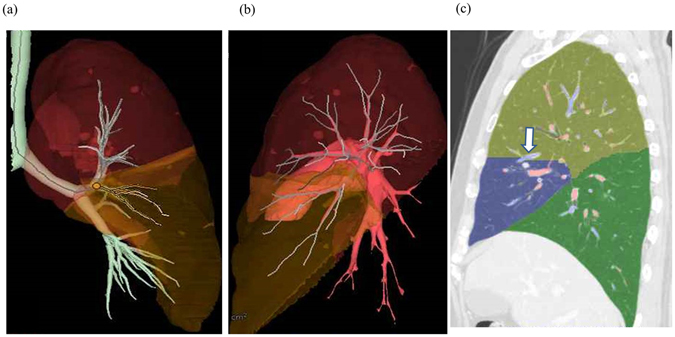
(a and b) According to the 3D-CT images, lingular bronchi (B4 + 5) and segmental arteries (A4 + A5) are identified. Subsequently, the perfusion area is depicted using an algorithm based on the direction and diameter of the bronchi and artery. (c) Visualization of intersegmental plane defined by intersegmental veins (V3a and V3b).
